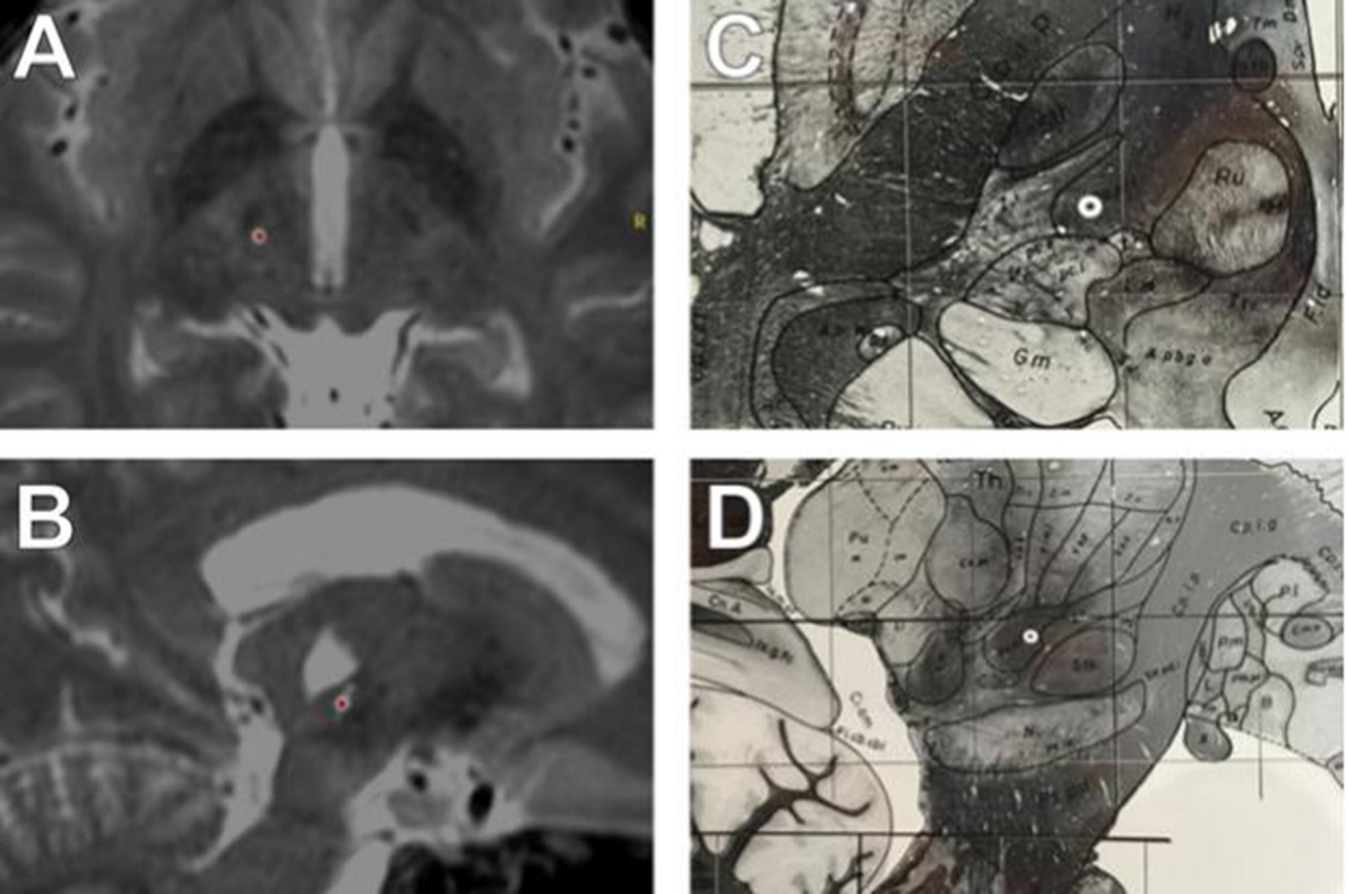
T2 MRI of electrode implantation site, axial (A) and sagittal (B) views. Target location on the Schaltenbrand and Wahren atlas (12) in axial (C) and saggital (D) views. Schaltenbrand G, Wahren W (12) Atlas for stereotaxy of the human brain. Stuttgart: Thieme. T2-SPACE MRI, merged with intraoperative CT imaging. (A–B). The electrode is marked with a red dot. AC/PC coordinates were (Lat: −11, A-P: −5, Vert: −1.5). (A) Axial view, contact 1. Note STN is visible anterior to electrode location, with analogous relative location noted in C. (B) Sagittal view, contact 1. Note the electrode location on the sagittal view relative to STN in B, and D. Note also the large cystic lesion superior to the electrode within the thalamus. Electrode location, Schaltenbrand and Wahren atlas, with electrode location marked based on AC/PC coordinates (white circle). (C) Electrode location in axial plane (Plate 54, H.v −1.5). (D) Electrode location in the sagittal plane Schaltenbrand and Wahren, (Plate 44, S.l. 13)
